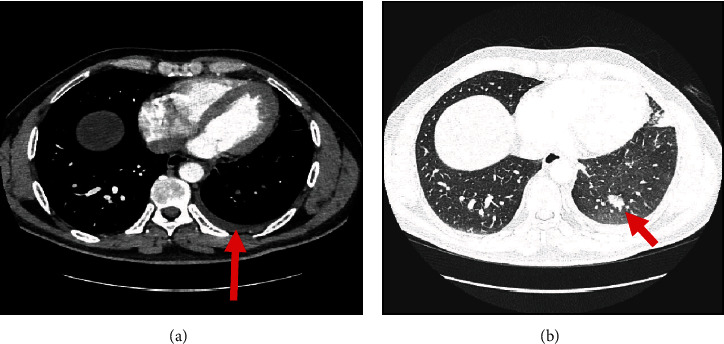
Computed tomography: (a) left pleural effusion (arrow); (b) consolidation and GGO in LUL (arrow). GGO: ground glass opacity; LUL: left upper lobe.
